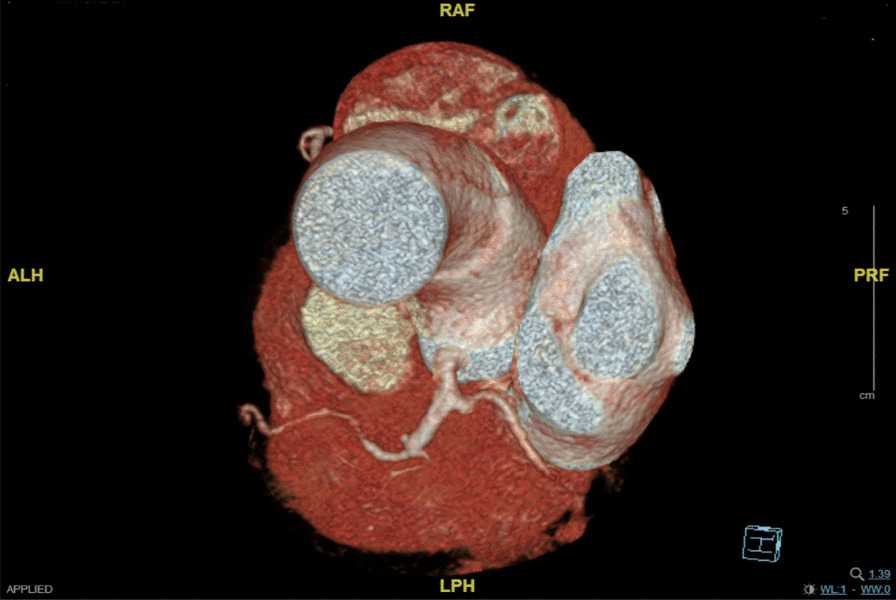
A reconstructed 3D coronary computed tomography angiogram image after the surgery showed successful correction of the lesions
